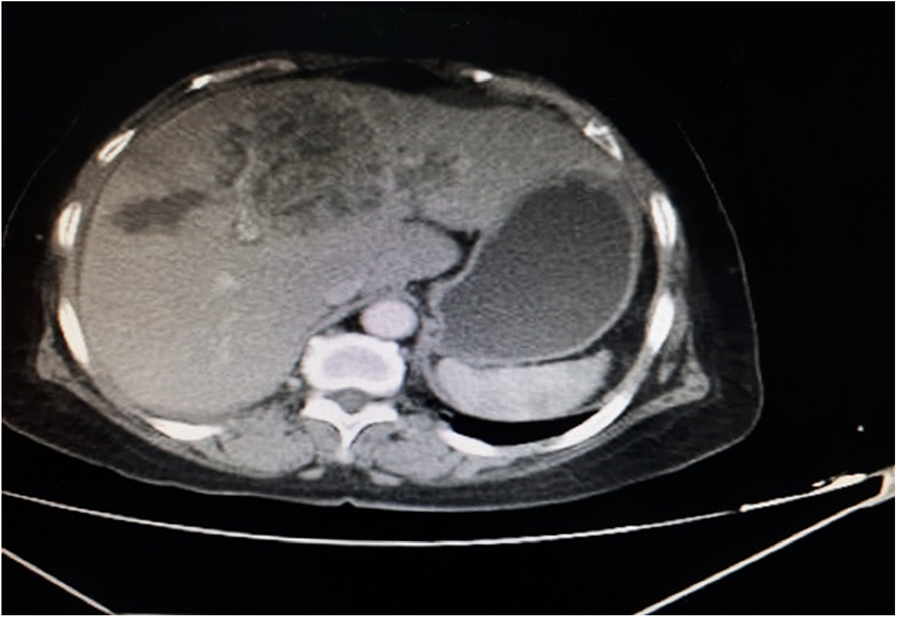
Abdominal computed tomographic scan with contrast revealing liver abscesses (*black arrows*) due to *Edwardsiella tarda* infection
